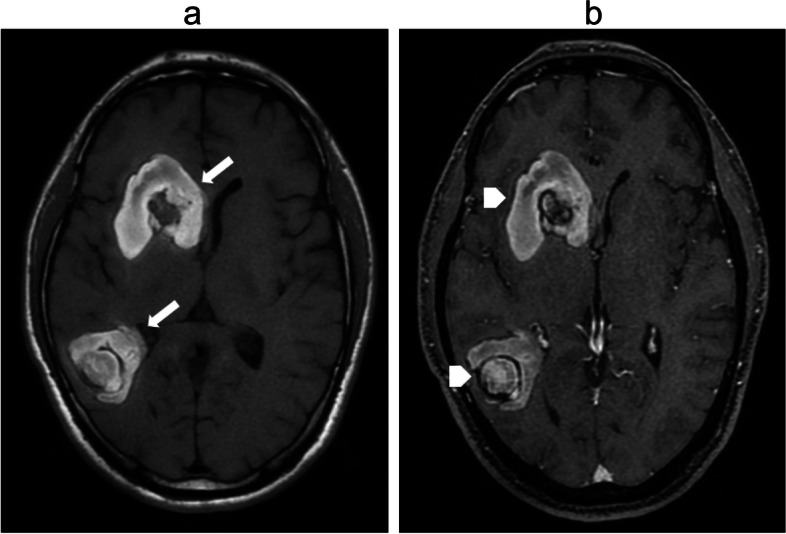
Contrast-enhanced MRI revealed metastatic brain lesions in symptomatic patients (a): Lesions at the frontal, right temporal, and lateral ventricles with predominantly high signal intensity on T1-weighted image (arrows). b Contrast MRI enhancement strongly infiltrated the centers of the lesions (arrow heads)
